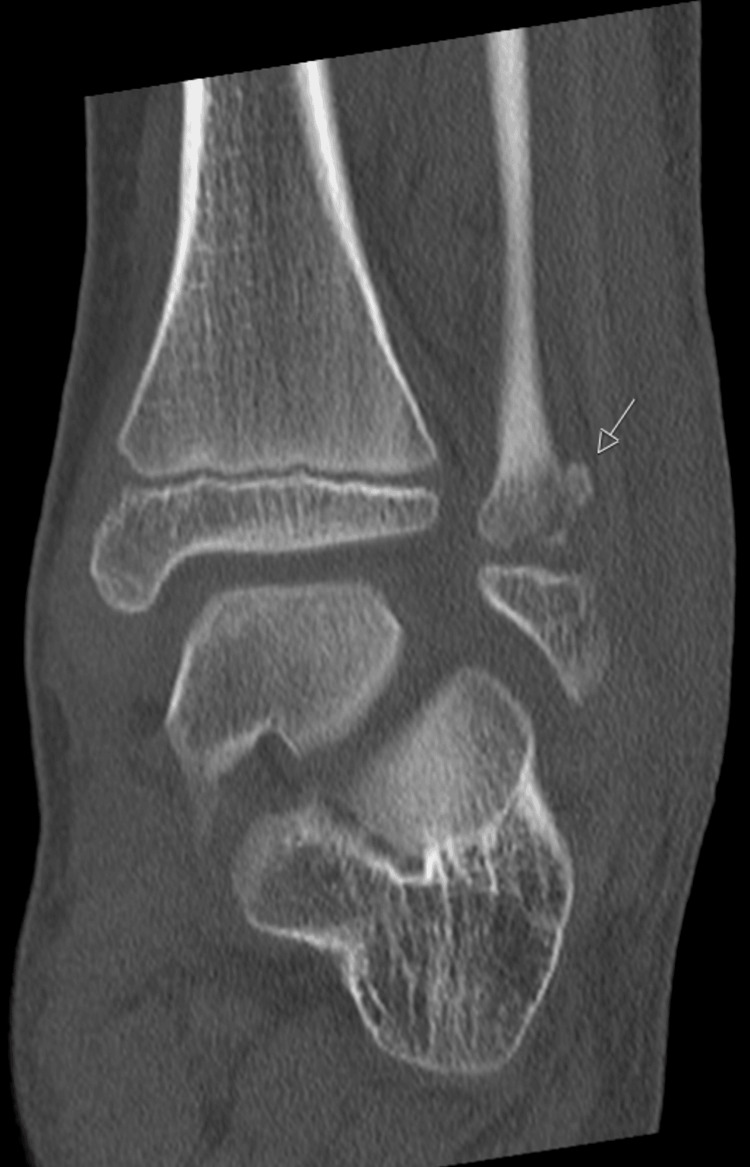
An AP projection of the CT scan, with the arrow pointing the metaphyseal fragment of the distal fibular epiphysiolisthesis.
